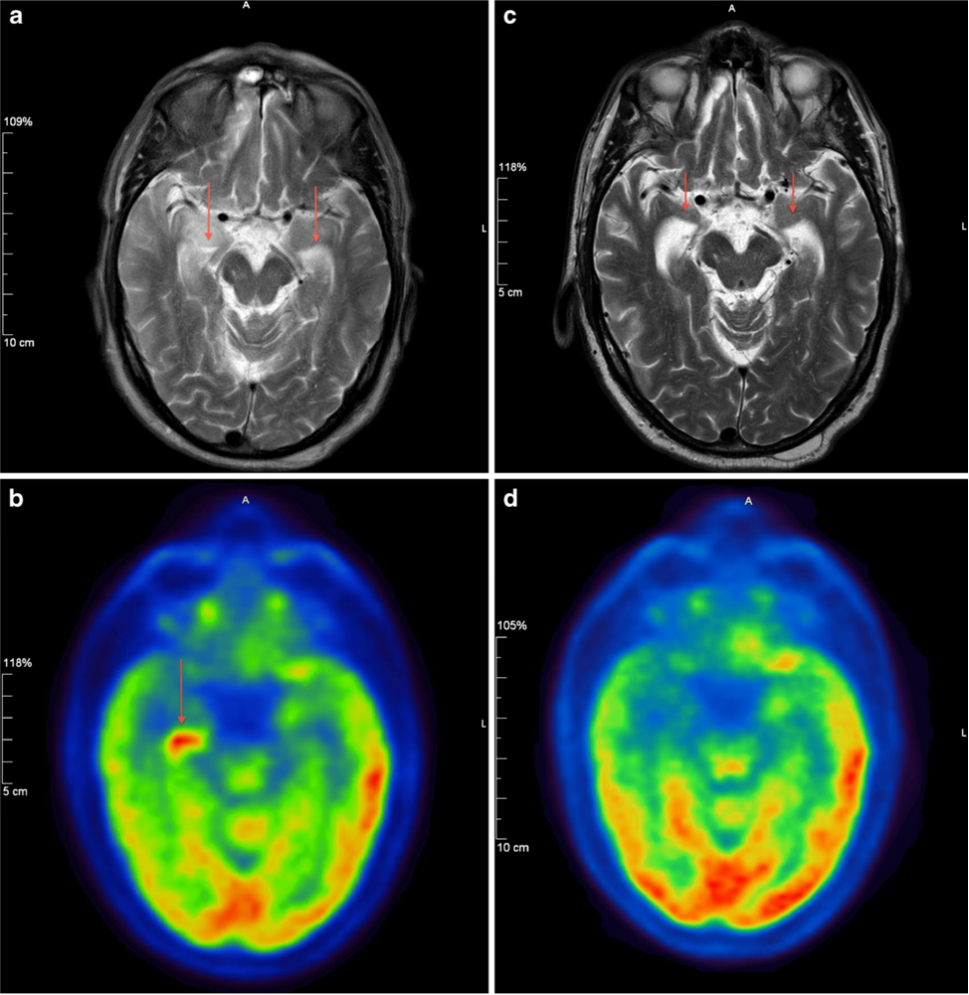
Brain imaging studies. (a) Pre-treatment brain magnetic resonance imaging scan showing bilateral mesial temporal high T2 signal intensity (arrows). (b) Pre-treatment brain positron emission tomography/computed tomography brain scan revealing a focus of intensely increased 18F-fluorodeoxyglucose uptake limited to the head of the right hippocampus (arrow) on a background of globally decreased 18F-fluorodeoxyglucose uptake. (c) Post-treatment brain magnetic resonance imaging scan showing marked improvement in the previously identified bilateral hyperintensities, which were replaced by atrophy (arrows). (d) Post-treatment positron emission tomography brain scan showing normal 18F-fluorodeoxyglucose uptake in the right hippocampus.
